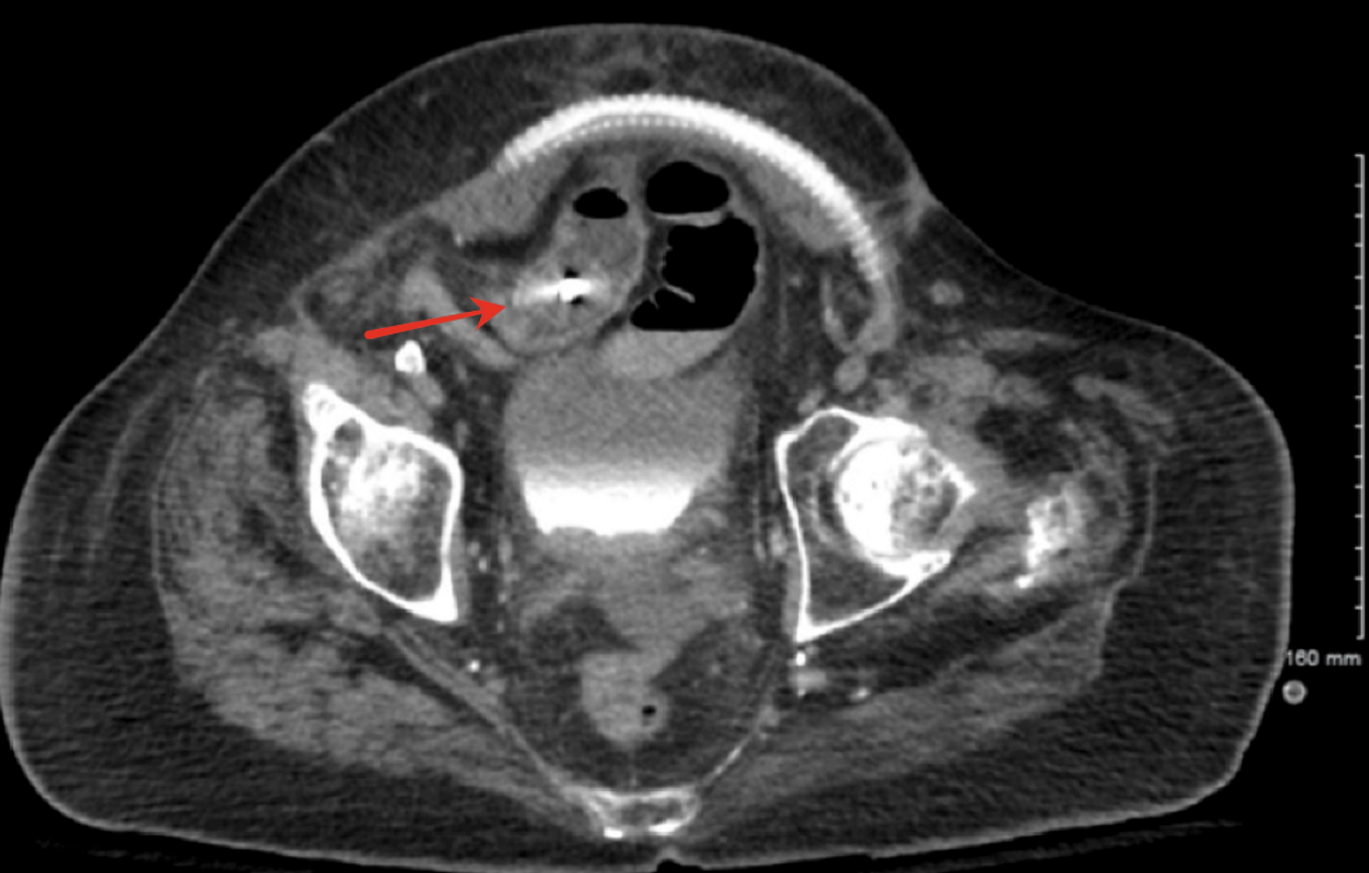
Axial CT image of the abdomen and pelvis with oral contrast demonstrating a calcified intraluminal gallstone causing small bowel obstruction (transition point indicated)
